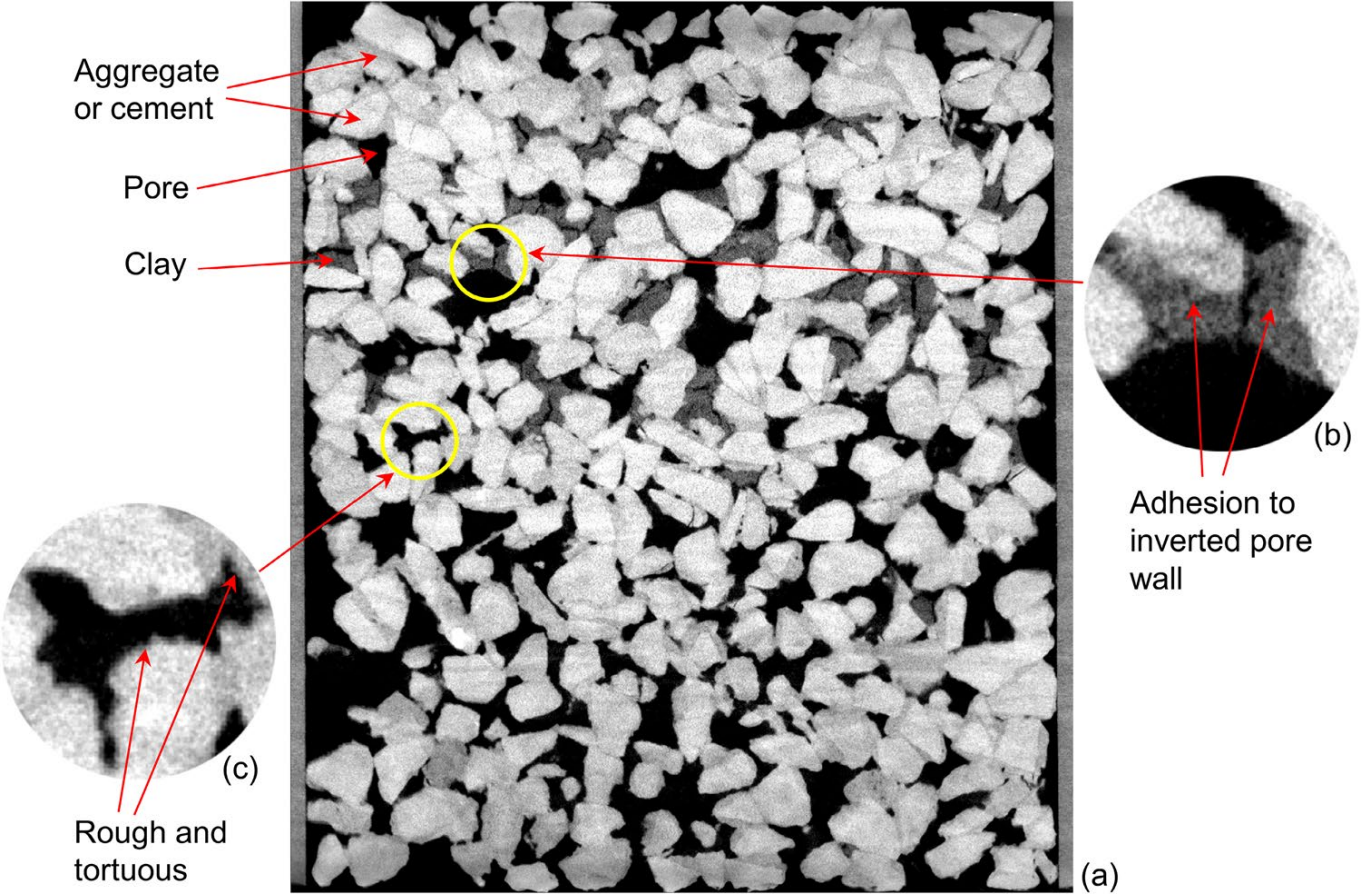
The CT image of the dried clay-clogged sample after all 30 washing cycles: (a) vertical image; (b) particles adhering to inverted pore wall; and (c) rough tortuous pore wall.
